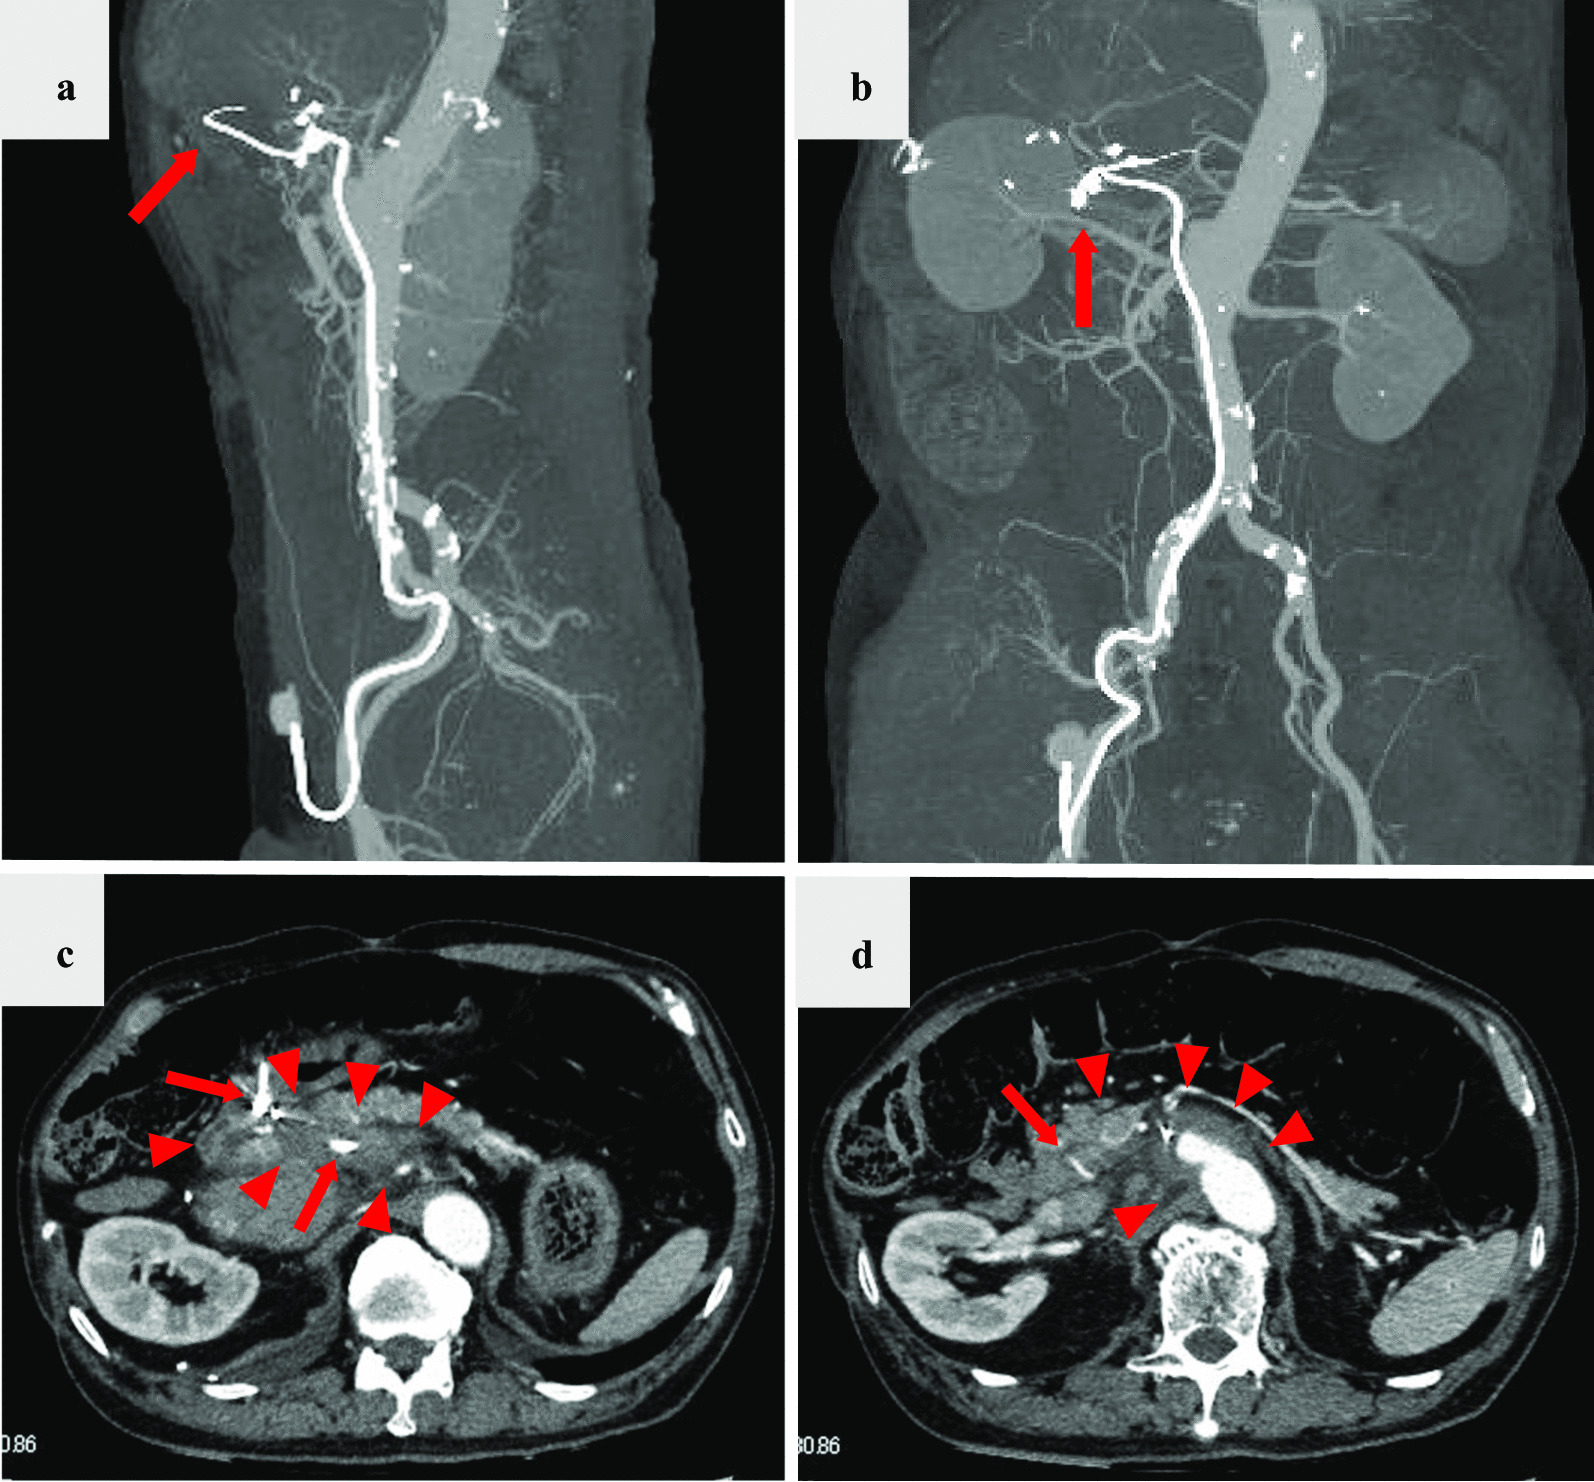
Computed tomography of the chest and abdomen reveals the thoracoabdominal aortic aneurysm along with the implanted arterial catheter inserted from the left femoral artery to the hepatic artery (a, b arrow). An irregularly shaped aortic aneurysm was identified at the origin of the celiac artery, with partially expanded common hepatic artery with disproportionate fat stranding (c, d arrowhead) along the catheter (c, d arrow); no extravasation was observed using contrast enhancement (c, d)
